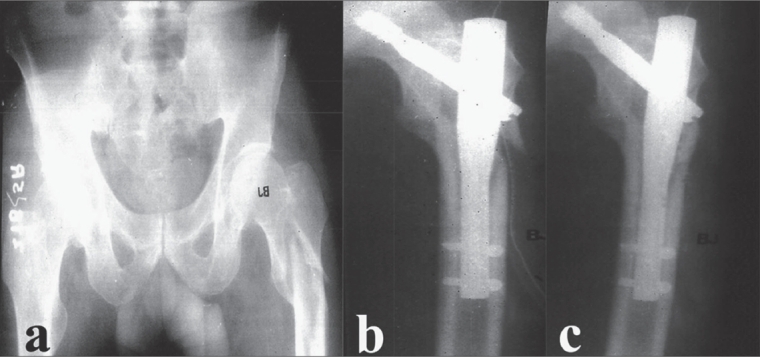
Radiograph showing comminuted trochanteric fracture (A3/AO classification) left femur. (b) Immediate postoperative radiograph showing good fracture alignment and reduction with gamma nail. (c) 1 year follow up showing good union at fracture site
